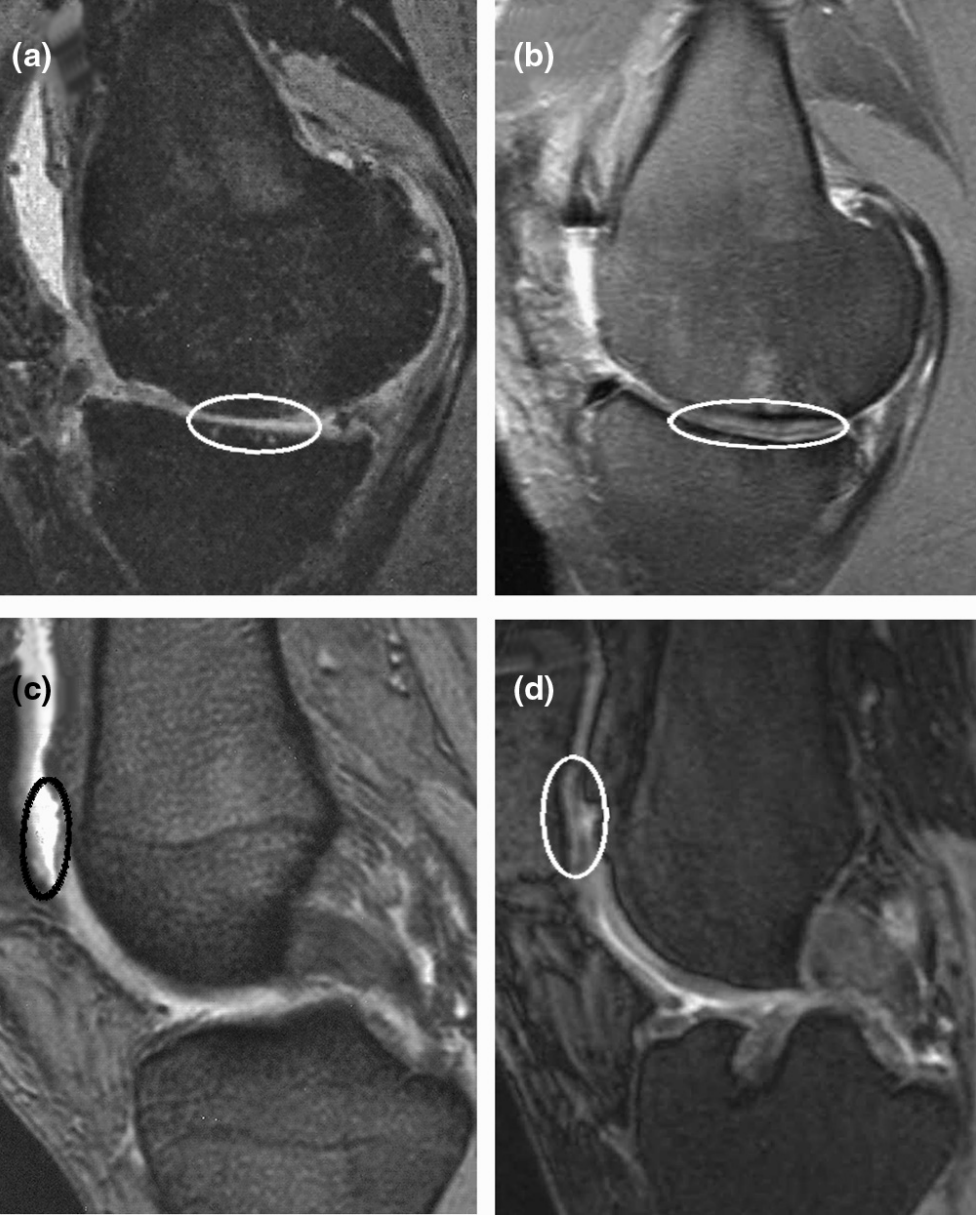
Magnetic resonance imaging (MRI) before and four years after implantation of BioSeed^®^-C. (a) Preoperative MRI shows a cartilage defect (encircled) at the medial femoral condyle. (b) After four years, MRI documented complete filling of the defect. Preoperatively, MRI shows a patellar (c) cartilage defect (encircled) that was completely filled after implantation of the graft as assessed by MRI at four years (d).
